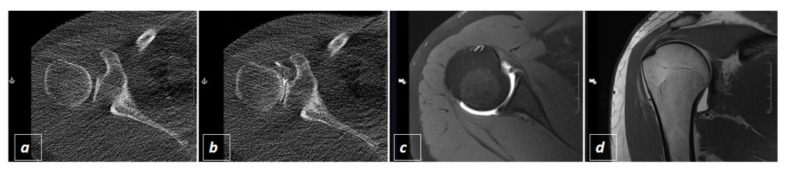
Example of CT-guided shoulder injection displaying corresponding transversal slices of the glenohumeral joint before (a) and after (b) CT-guided application of Iopamidol. Subsequent visualization of intraarticular application of Gadopentetat-Dimeglumin resulting in good joint distension as demonstrated in the following MR-arthrography in transversal (c) and coronal (d) T1 weighted images with (c) and without (d) fat-saturation.
